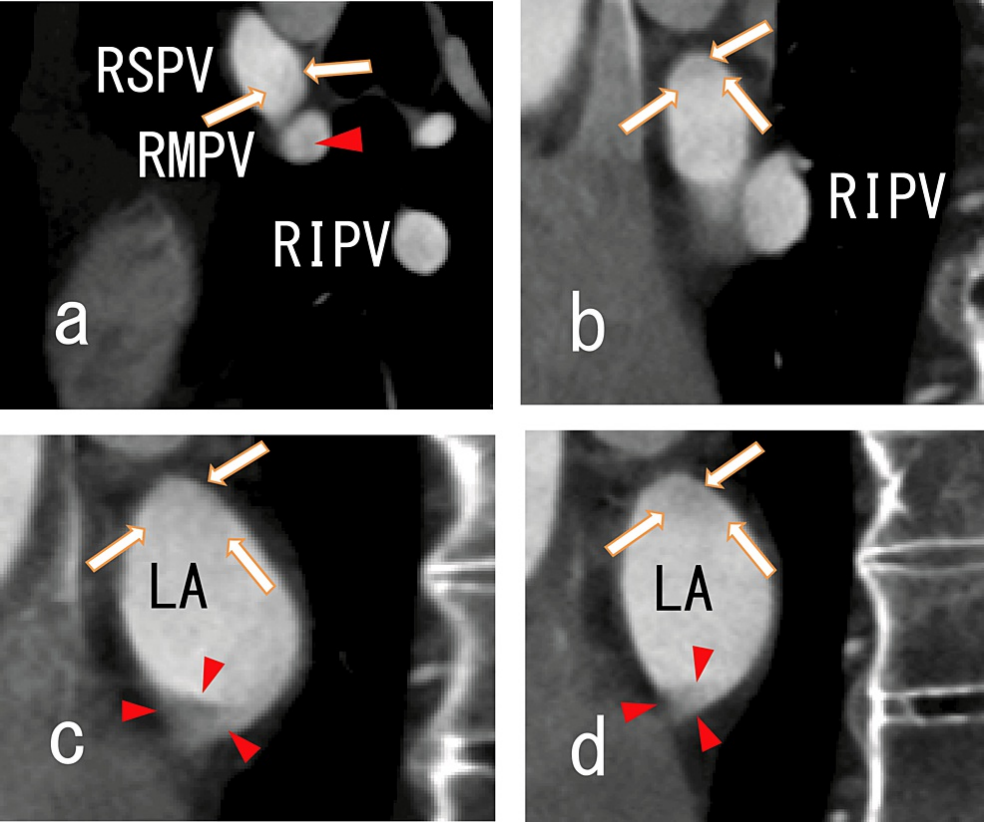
Sagittal images from an 80-MDCT scan obtained after one month of standard-dose heparin-warfarin remedy showing thrombi in the RSPV, RMPV, RIPV and LA (a) There were rather dark areas in the RSPV (arrows), suggesting that thrombi were partly resolved. There were rather dark areas in the RMPV (arrowhead), suggesting that there were thrombi in the RMPV. There were dim dark areas in the RIPV. (b) After the RSPV’s connection to the RMPV, the RSPV then linked to the RIPV. There were rather dark areas in the anterosuperior region of the RSPV (arrows), suggesting that there were thrombi in the RSPV. There were dim dark areas in the RIPV. (c) There were thin, vague, dark areas in the superior region of the LA (arrows), suggesting that the thrombi in the LA had partially resolved. There were new dark areas in the inferior region of the LA (arrowheads). (d) There were rather dark areas in the posterosuperior region of the LA (arrows), suggesting that there were thrombi in the LA. The merging of the thrombi was vague. There were new dark areas in the inferior region of the LA (arrowheads). LA, left atrium; RIPV, right inferior pulmonary vein; RMPV, right middle pulmonary vein; RSPV, right superior pulmonary vein; 80-MDCT, 80-slice multidetector computed tomography
